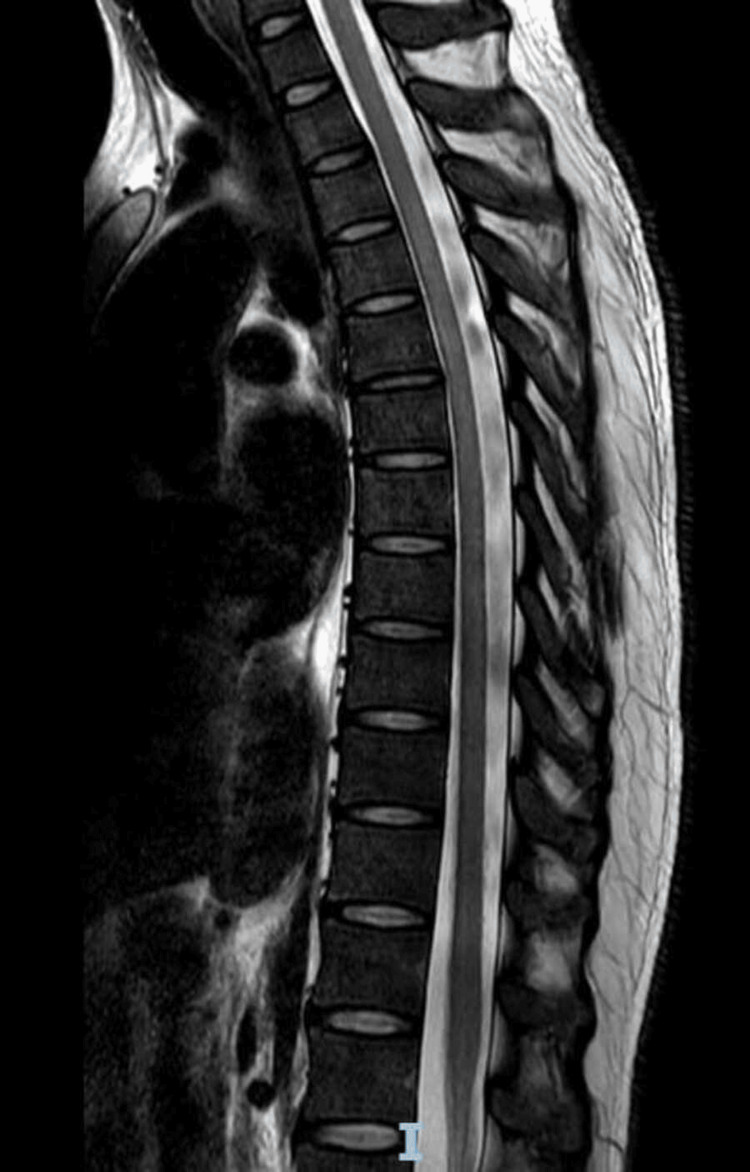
MRI thoracic spine (Sagittal view) - Normal marrow signal and no other significant abnormality
